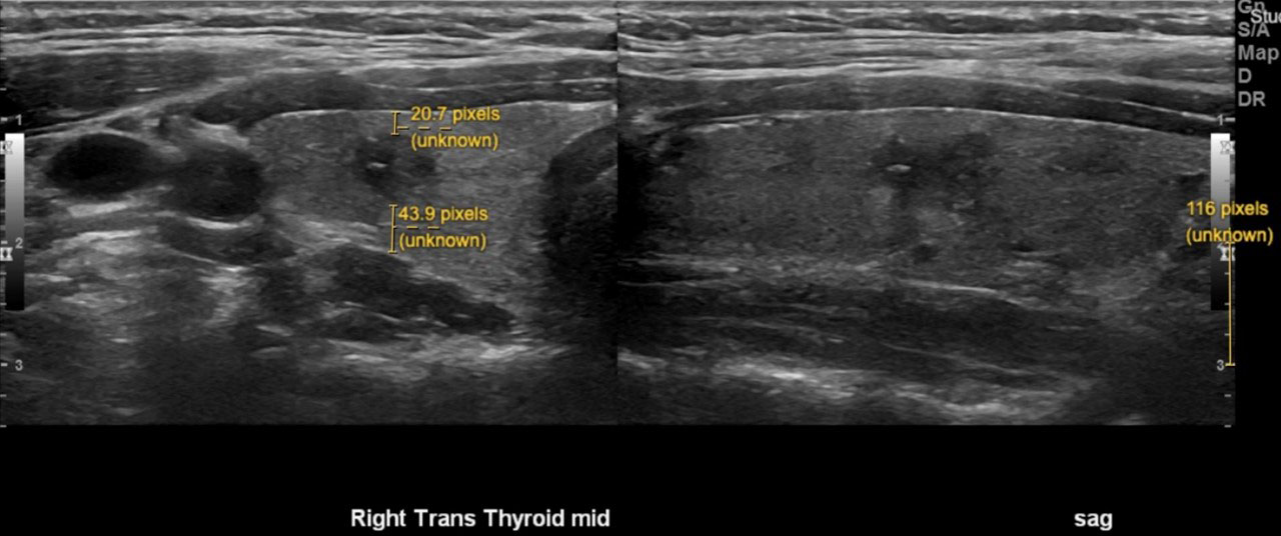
Representative image demonstrating preoperative ultrasound measurements of thyroid nodules. Size of nodule (white). Distance to anterior and posterior capsule (yellow). The pixel size was calibrated for each image using the scale provided by the ultrasound software (bottom right of image).
